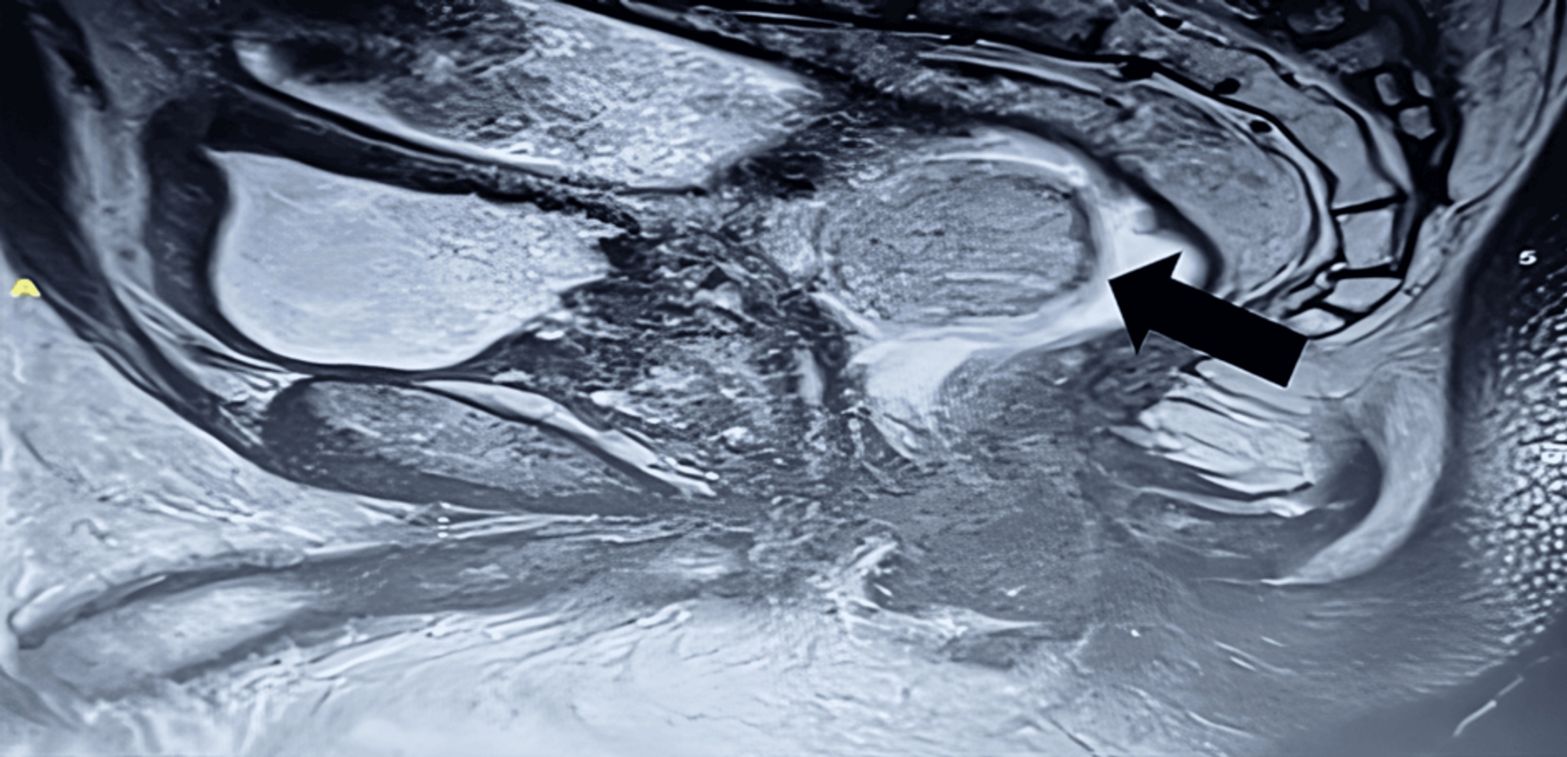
An MRI image shows that the tumor was located in the mid-rectal mass, 7.6 cm from the anal verge, measuring 3.5 x 4 x 3.4 cm, with intermediate T2 and high T1 signal intensity (arrow). MRI, magnetic resonance imaging
